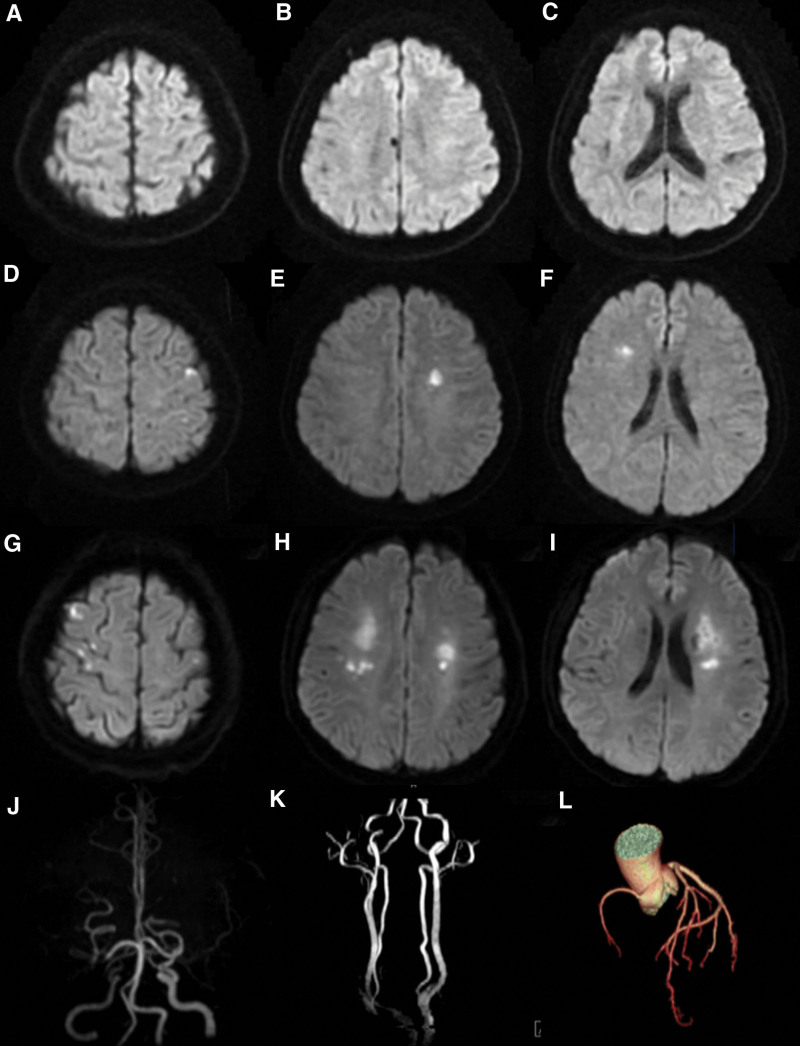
The first brain magnetic resonance imaging shows no acute cerebral infarction on diffusion-weighted imaging (A, B and C), whereas magnetic resonance angiography shows occlusion of the bilateral middle cerebral arteries (J) but no occlusion of the carotid arteries (K). After the second episode multiple acute cerebral infarctions appeared in both hemispheres (D, E and F), whereas several new lesions appeared after the third episode (G,H and I). Coronary angiography showed normal results (L).
